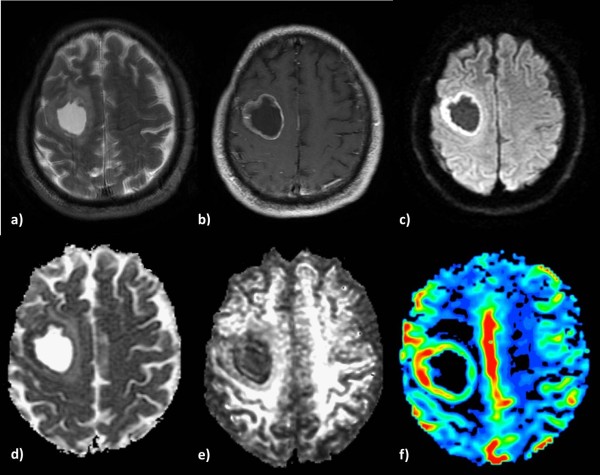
Intracranial lung metastasis in a 68 year-old-man. a) T2-weighted image, b) ring-shaped enhancement on a T1-weighted post contrast edema, c) restricted diffusion in the periphery of the tumor. Increased intratumoral ADC (d), decreased FA (e) and elevated perfusion in the peripheral solid part of the lesion (f).
